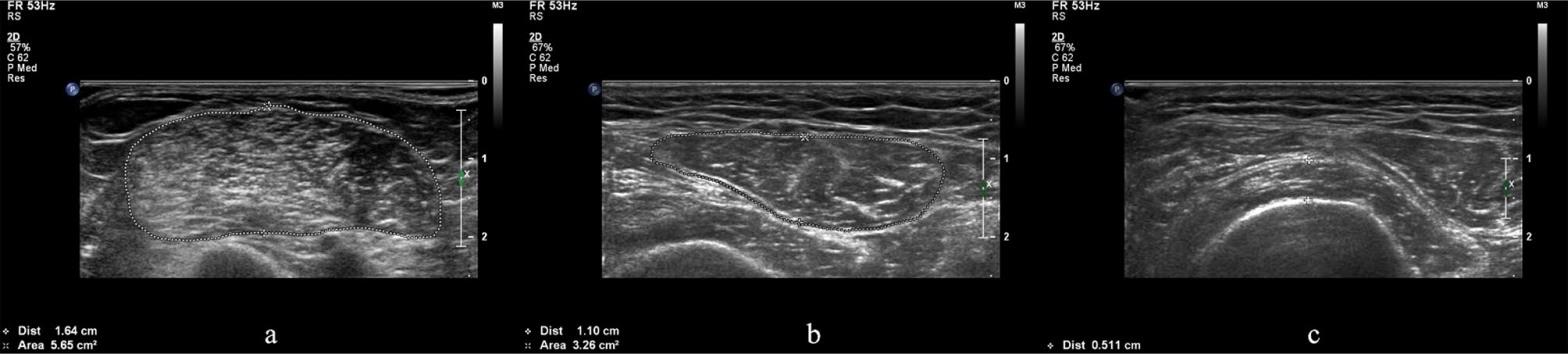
Ultrasound appearance of muscle thickness and cross-sectional area. Appearance and ultrasound measurement of thickness and cross-sectional area of biceps brachii muscle (a), rectus femoris muscle (b), and vastus intermedius muscle (c).
